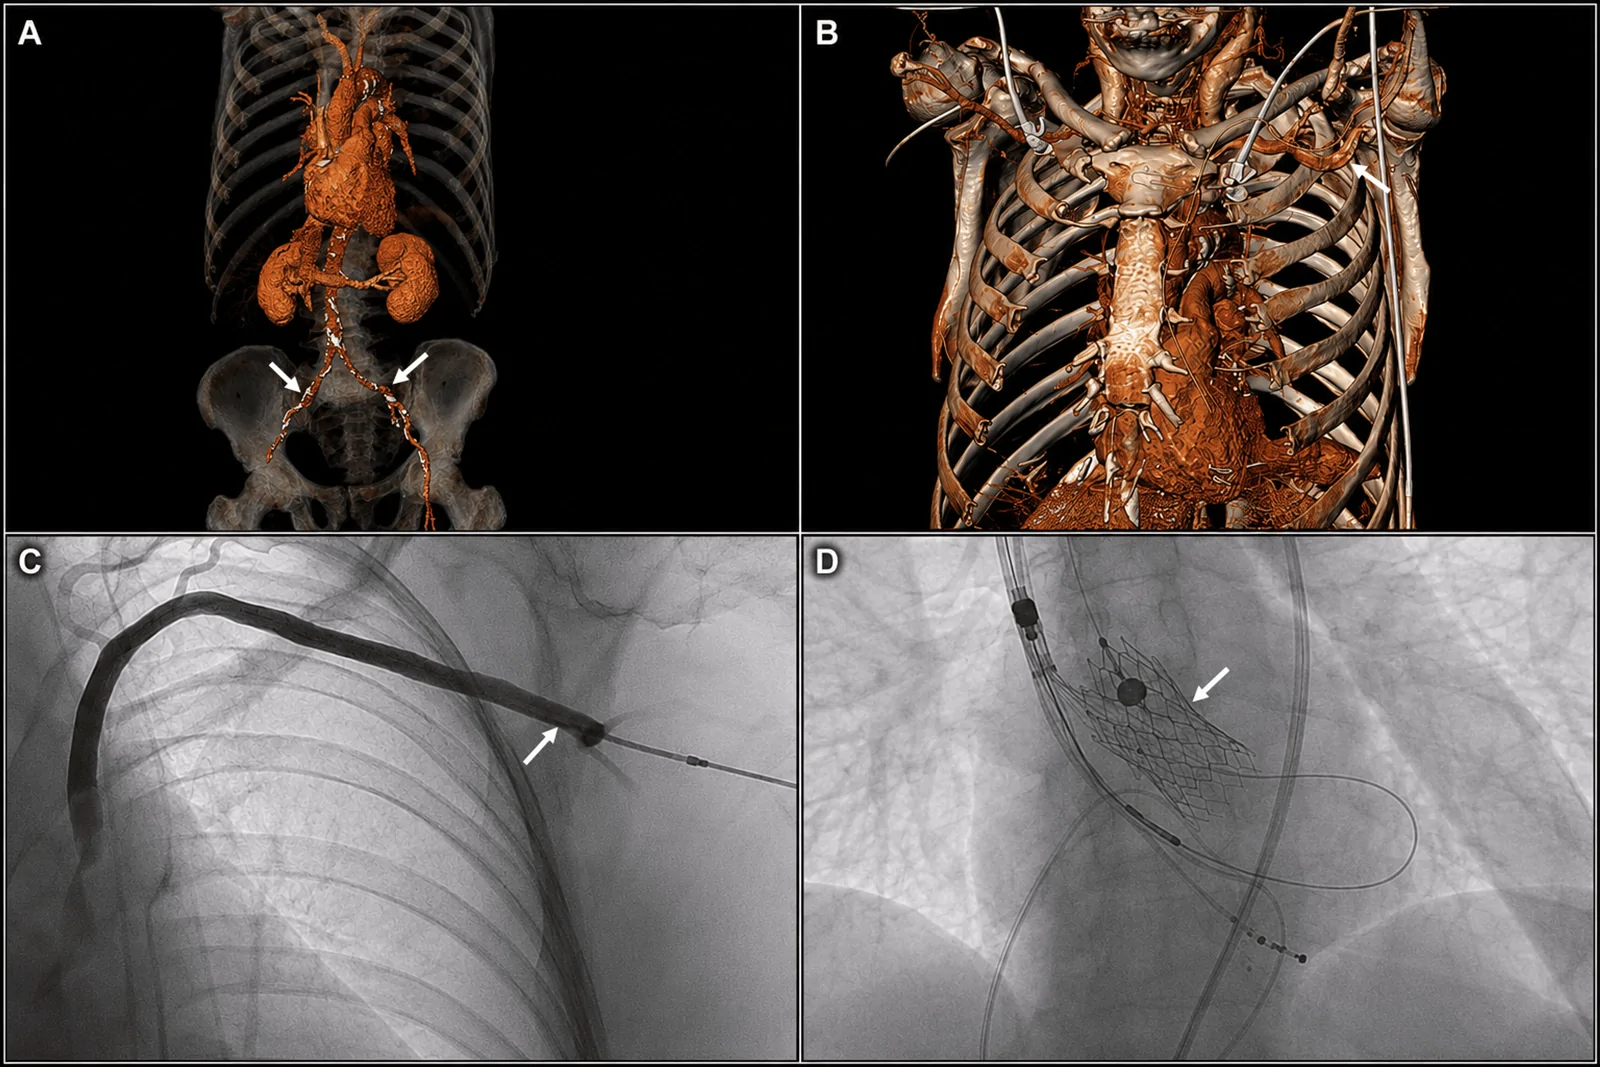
Anatomical evaluation and implantation of a transcatheter aortic valve through a left transaxillary approach. (A) Three-dimensional reconstruction of preprocedural computed tomography angiography demonstrating extensive vascular disease and unsuitable transfemoral access (arrows). (B) Three-dimensional reconstruction of preprocedural computed tomography angiography showing the selected left transaxillary approach (arrow). (C) Fluoroscopic image showing the left transaxillary access route (arrow) used for TAVR. (D) Final fluoroscopic view after implantation of the 27-mm Navitor^TM^ Vision aortic valve (Abbott Vascular, Santa Clara, USA) (arrow). TAVR: transcatheter aortic valve replacement
